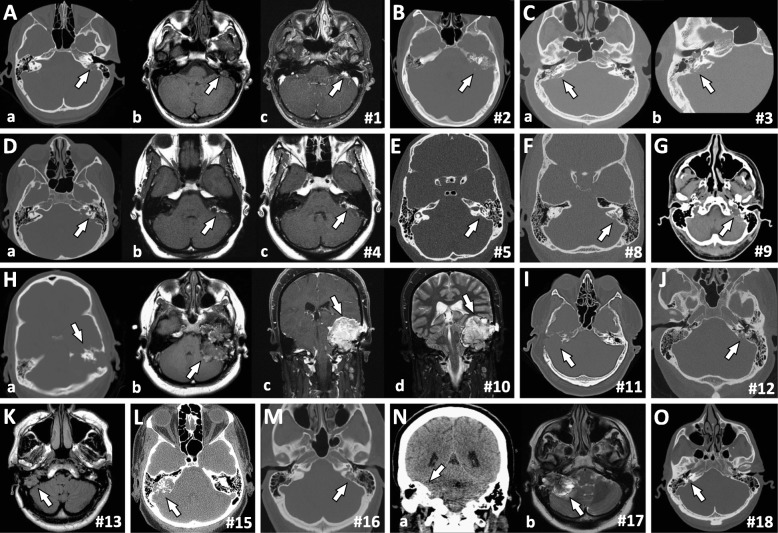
Imaging Findings: Computed tomography shows bone destructive lesions involving the posterior aspect of the petrous temporal bones (Aa, B, C, Da, E-G, Ha, I, J, L, M, Na, O). Magnetic resonance imaging shows expansile complex partially solid and cystic masses centered on the petrous segment of the temporal bones demonstrating heterogeneous intrinsic T1 (Ab, Db, Hb) and T2 signal (Hd, Nb) and post-contrast enhancement (Ac, Dc, Hc). (# represents case number). Note: All images are pre-operative except K which is an axial T1-weighted post-operative (at recurrence) image
